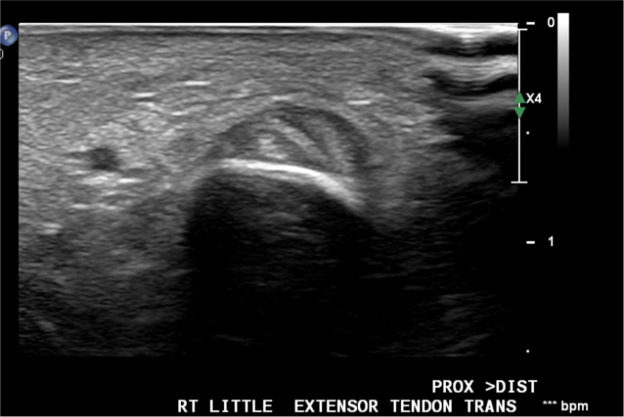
Ultrasound of flexor tendons (transverse, Case 1).
